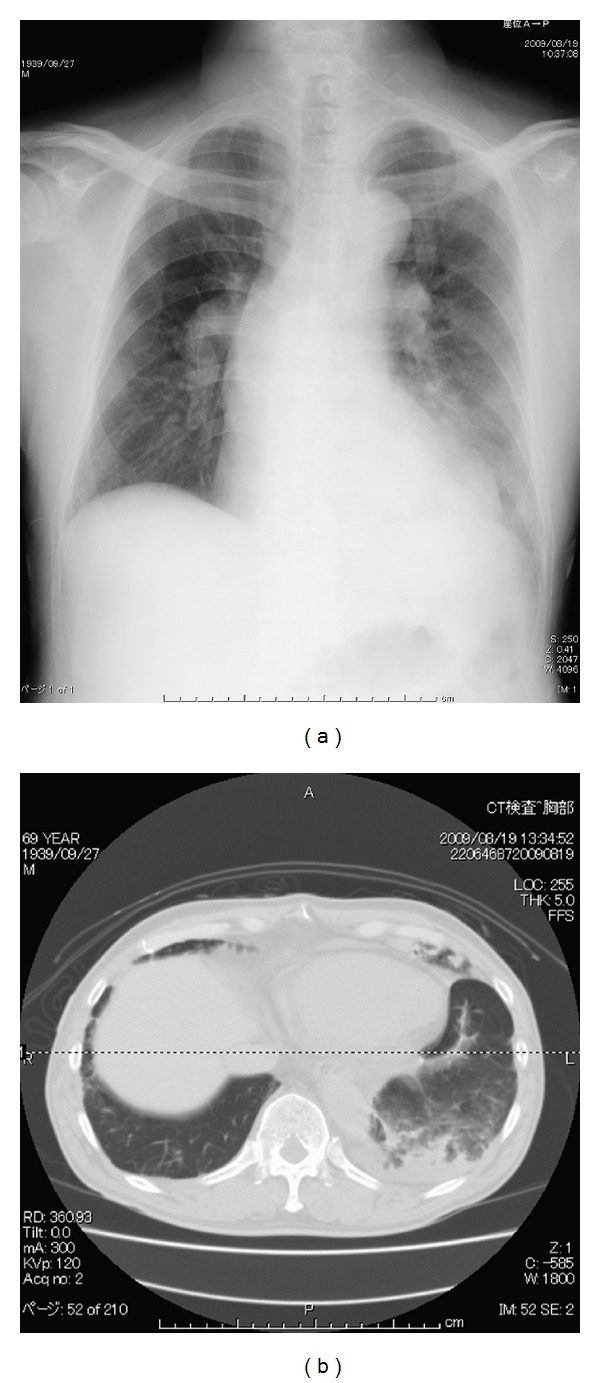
Chest X-ray and representative computed tomography image obtained on day 1. The patient had localized consolidation on his left lower lobe, suggesting postsurgical pulmonary edema in the dilated segment.
